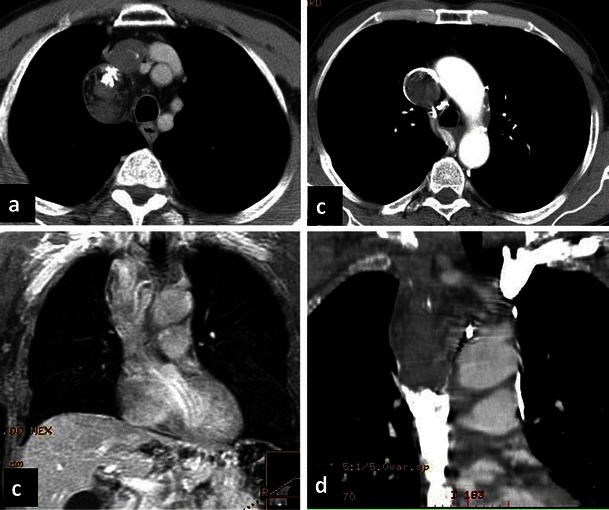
Teratoma. Non-contrast and contrast-enhanced CT (a, b) shows a rounded nodule, with some intralesional fatty spots and calcifications in the right paratracheal space consistent with teratoma. The benign lesion induced extrinsic compression of the superior vena cava
